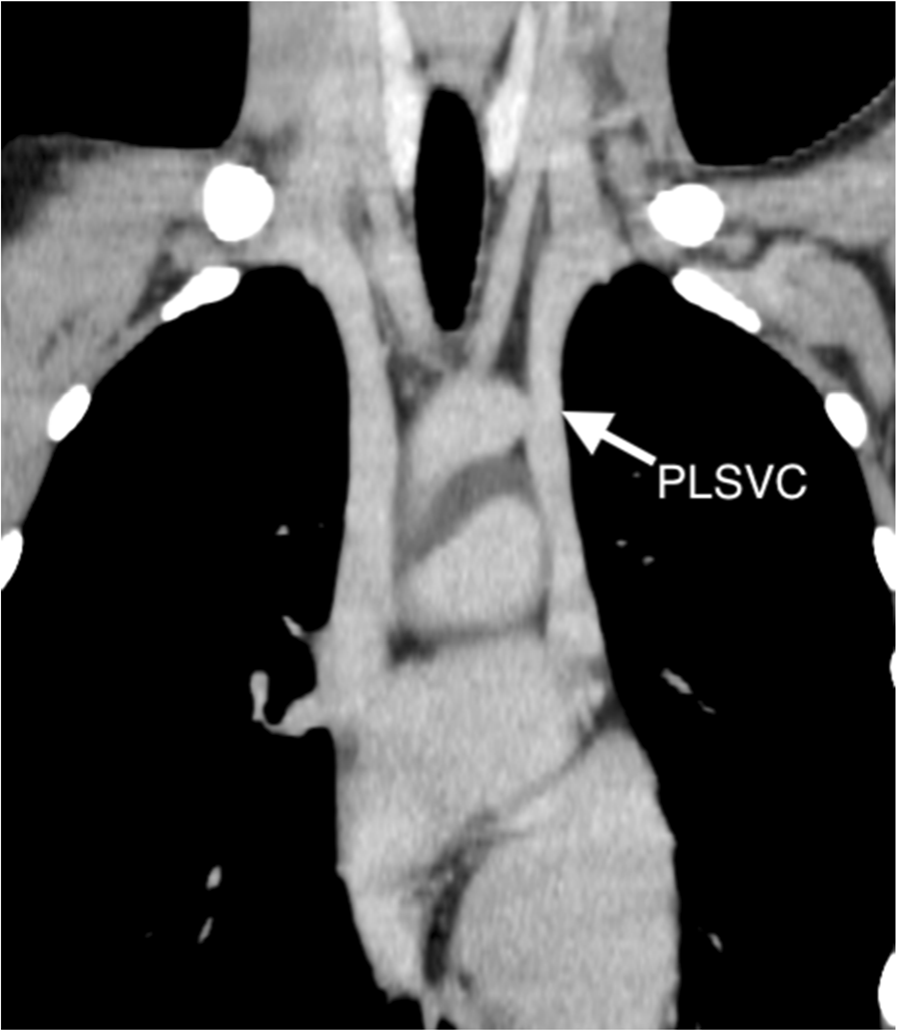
Preoperative computed tomography shows persistent left superior vena cava (PLSVC) and left brachiocephalic vein atresia
